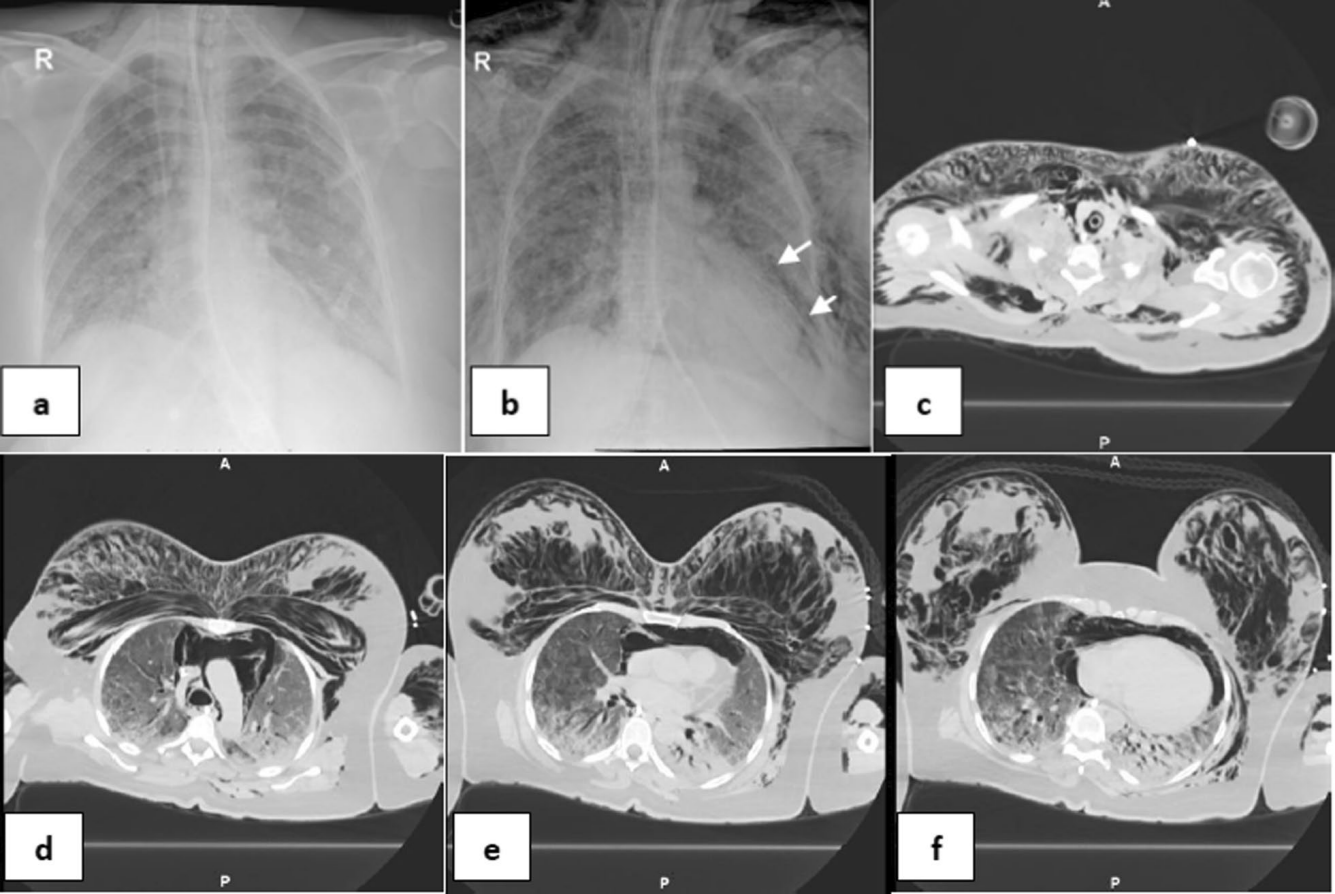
64-year-old female with hypertension and diabetes, intubated 3 days post admission. a, b Two serial frontal chest X-ray films demonstrate a pneumomediastinum 4 days after intubation (white arrows), and bilateral chest walls surgical emphysema. Note bilateral ill-defined air space opacities throughout both lungs more in left lower zone. c-f series axial CT cuts confirming the pneumomediastinum and bilateral extensive chest wall surgical emphysema
